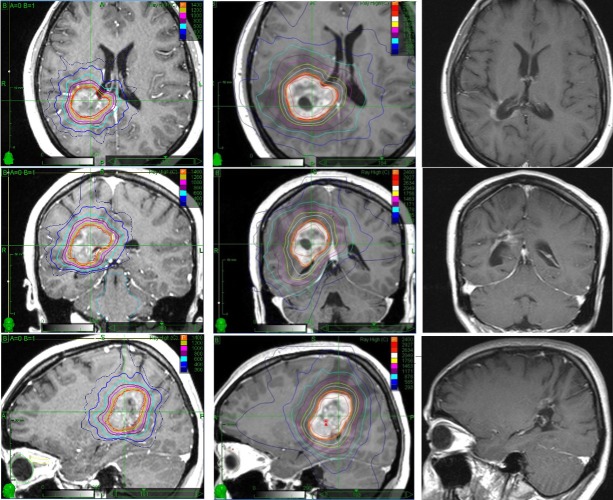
Axial, coronal, and sagittal T1-weighted (T1w) magnetic resonance images of the presented pregnant patient at the time of the first single fraction CyberKnife treatment (left column), at the time of the second fractionated CyberKnife treatment after delivery (middle column), and 43 months after delivery (right column). In the first and second column, the isodose distributions of the corresponding CyberKnife treatments are presented.
